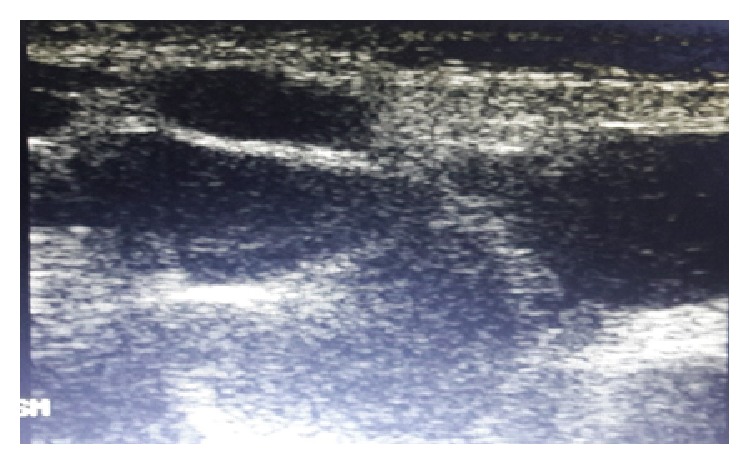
Ultrasound scan of the neck; multiple enlarged cervical lymph nodes at the levels of I-B, II, III, and IV. Largest at level II was 3.7 cm × 2.0 cm in size.
